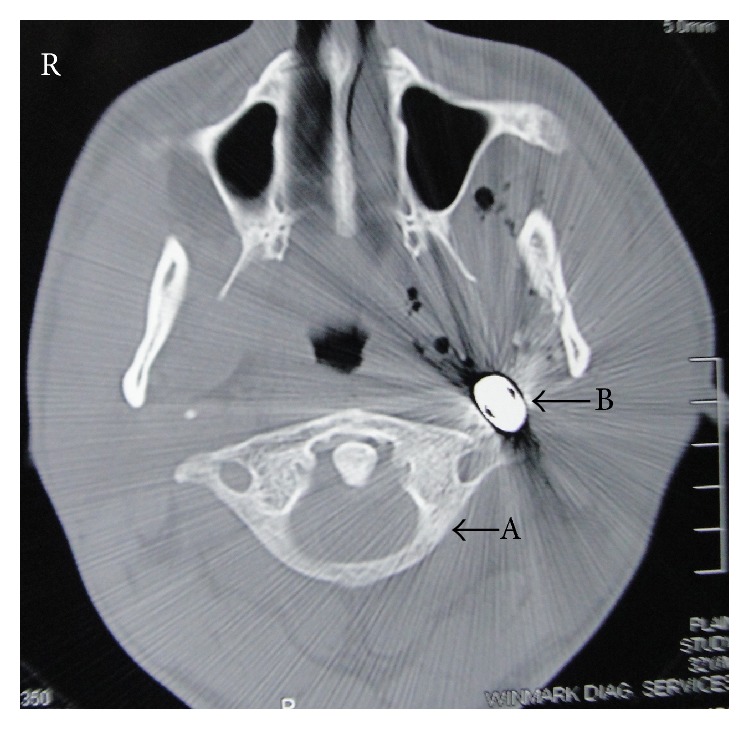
CT scan passing through the atlas, showing the bullet (B) in the left parapharyngeal space anterolateral to the transverse process of the atlas (A) with air pockets around it. Note the reflection of X-rays by the bullet.
